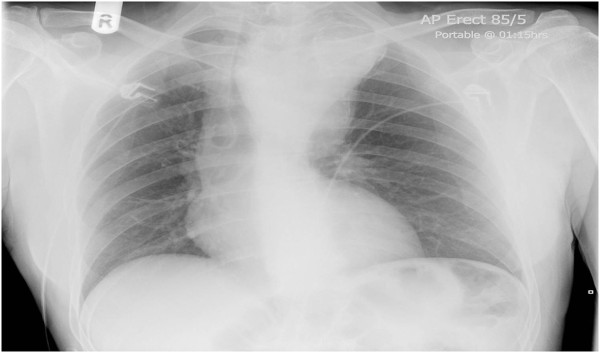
Chest X-ray showing a large superior mediastinal shadow suggestive of arch aneurysm extending well into neck with extreme tracheal deviation.
